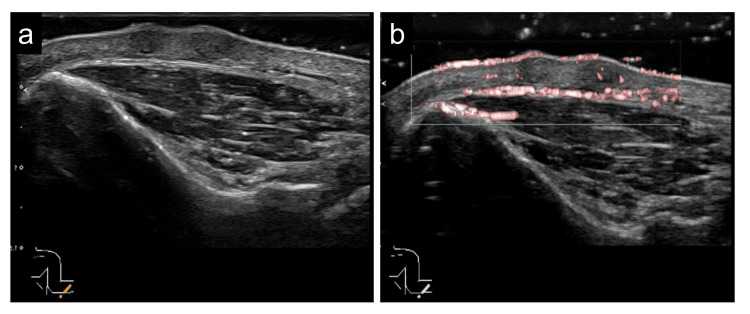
Echography findings (a) Echography shows a flattened, vaguely bordered, homogenous hypoechoic area. (b) Doppler imaging shows a hypoechoic area surrounded by an area of increased blood flow.
